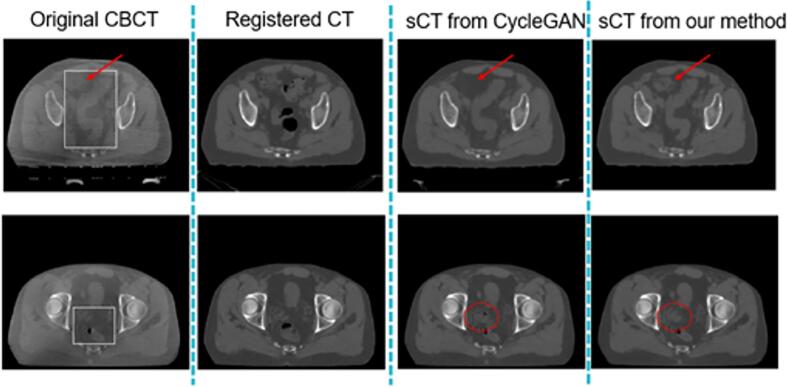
Examples comparing novel structure preservation for conventional cycleGAN and the improved cycleGAN with novel loss functions, both trained on the same datasets with same hyper-parameters. The white boxes indicate areas of structural mismatch between the original CBCT and the deformably registered CT. While the conventional cycleGAN may either remove (top row, red arrows) or produce (bottom row, red circles) structures on the original CBCT, the novel loss function from this work more consistently preserves the CBCT structures. (For interpretation of the references to colour in this figure legend, the reader is referred to the web version of this article.)
